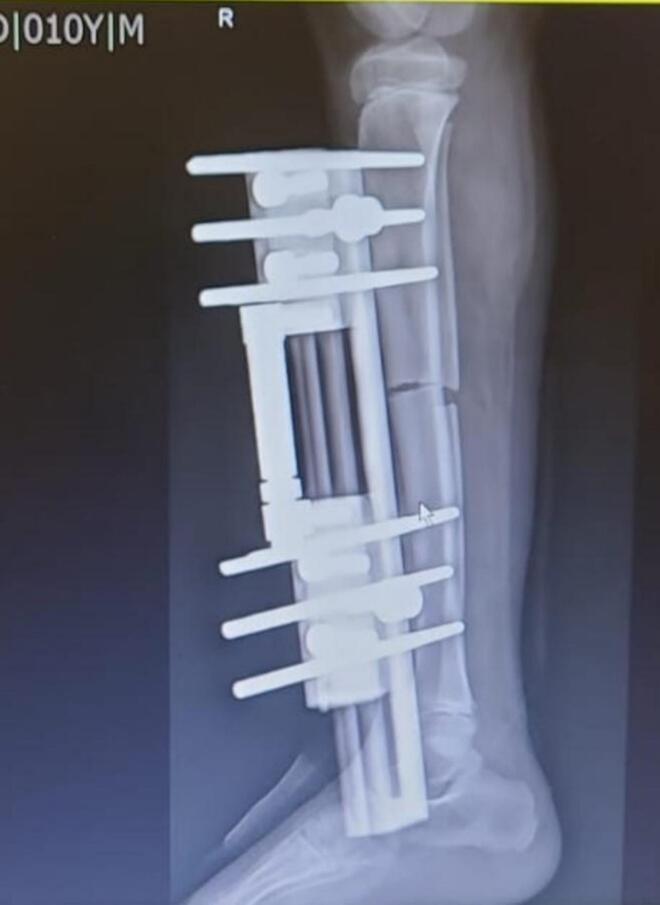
Xray Right leg (lateral view)- first post-operative day showing LRS fixator inserted with three Schanz pins each on either side of the mid diaphyseal corticotomy site of right tibia.
